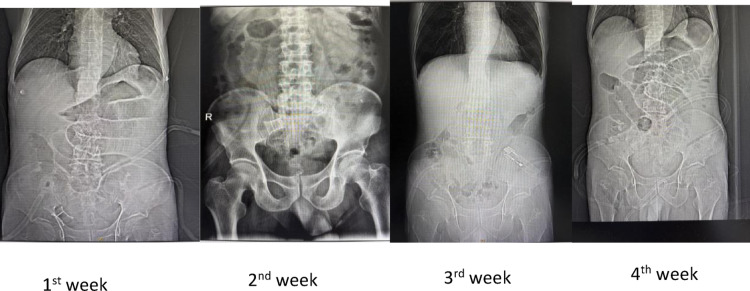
X-ray monitoring of stent degradation. The stents of the patient were degraded completely within 4 weeks
